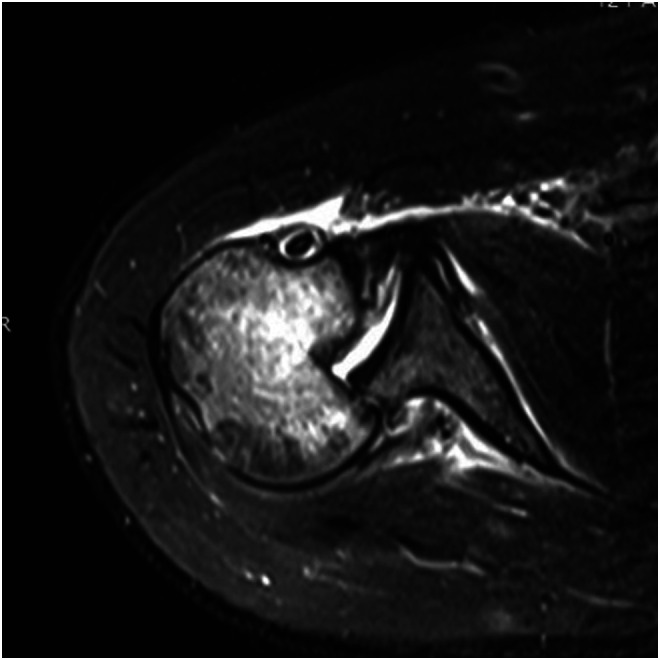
1st patient: MRI scan showing PSD, reverse Hill-Sachs, reverse Bankart lesion, intact posterior glenoid rim, intact lesser tubercle, and subscapularis tendon.
